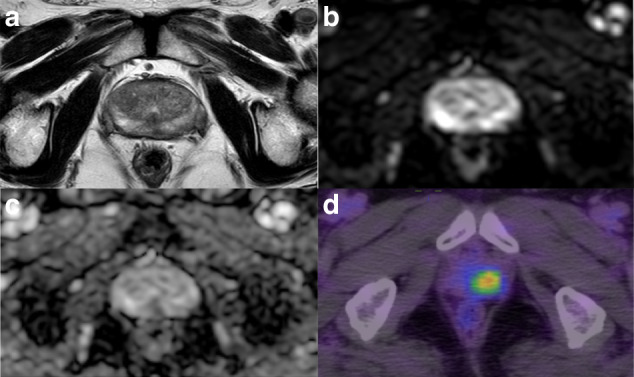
A positive lesion (SUVmax 14.3) on ^18^F-PSMA-1007 PET/CT was reported as PI-RADS 2 on mpMRI and confirmed to be prostate cancer on biopsy. (a) T2-weighted image, (b) diffusion-weighted image, (c) apparent diffusion coefficient map, and (d) ^18^F-PSMA-1007 PET/CT image.
